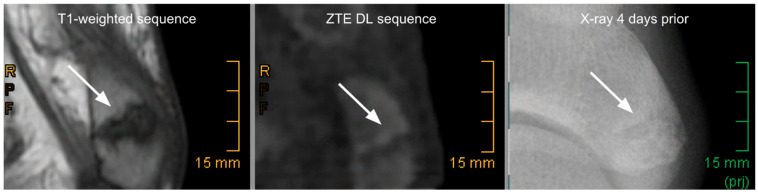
Eighteen-year-old male whose radiograph showed a suspected traumatic injury of the os naviculare, with os tibiale externum as a differential diagnosis, imaged with ZTE DL and T1-weighted MRI, with a corresponding radiograph obtained 4 days earlier. MRI with ZTE DL confirmed an os tibiale externum rather than a fracture.
